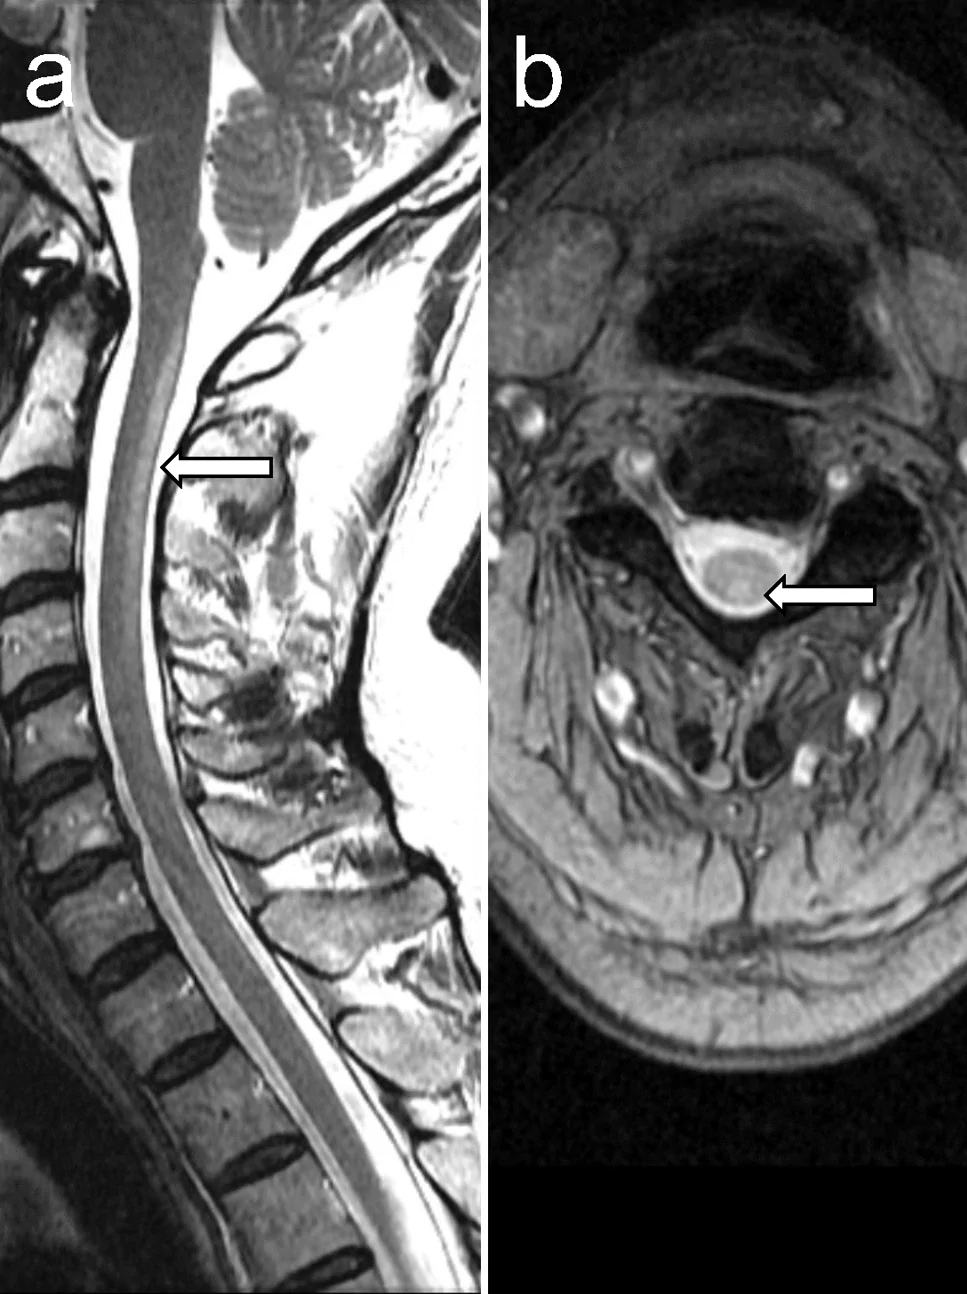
Neuroimaging case #9. Sagittal (a) and axial (b) T2-weighted image 21 months after LI showed a hyperintense lesion of the posterior funiculus ranging from C1 to 5
